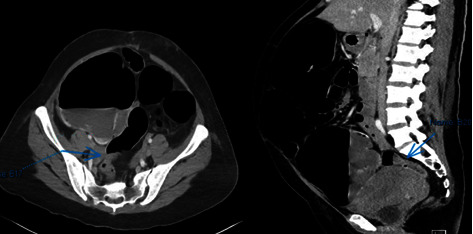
Whole abdominal CT scan with contrast. Description: large bowel obstruction with transition point at the rectosigmoid junction, likely from postoperative adhesions; pneumatosis coli in the cecum and ascending colon; no ascites, pneumoperitoneum, or enlarged lymph nodes; prominent-sized appendix without inflammatory changes; and anteverted and globularly enlarged uterus with a prominent endometrium.
